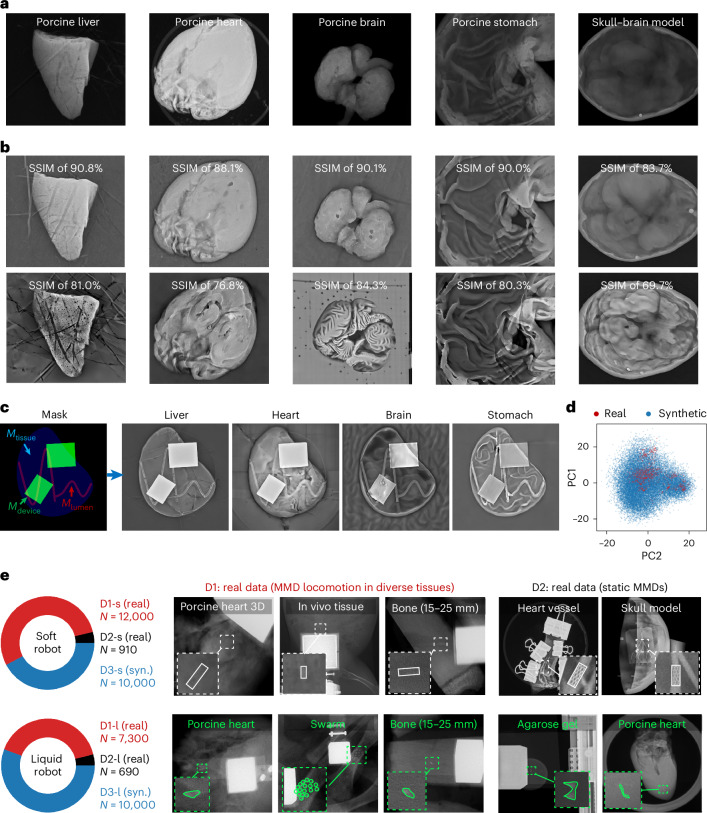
Domain randomization of synthetic tissue images and open-sourced MMD X-ray dataset. a, Real tissue images under X-ray imaging. b, The generation of precisely matched synthetic tissues with randomized and enhanced textures. c, Image generation with mask-guided conditioning and prompts. Randomization of masks and prompts substantially expands the dataset. d, A domain comparison of real and synthetic tissues. Features from 1,140 real and 24,803 synthetic tissue images, extracted using Inception V3, are visualized via PCA. e, An overview of the open-source MMD dataset under X-ray imaging. Dataset 1 (D1) contains real-time recordings and annotations of MMD locomotion. Dataset 2 (D2) includes static MMD images captured under various voltage and current conditions. Dataset 3 (D3) provides synthetic images with corresponding segmentation labels. The suffixes ‘-s’ and ‘-l’ denote the soft and liquid MMDs, respectively. Source data.
